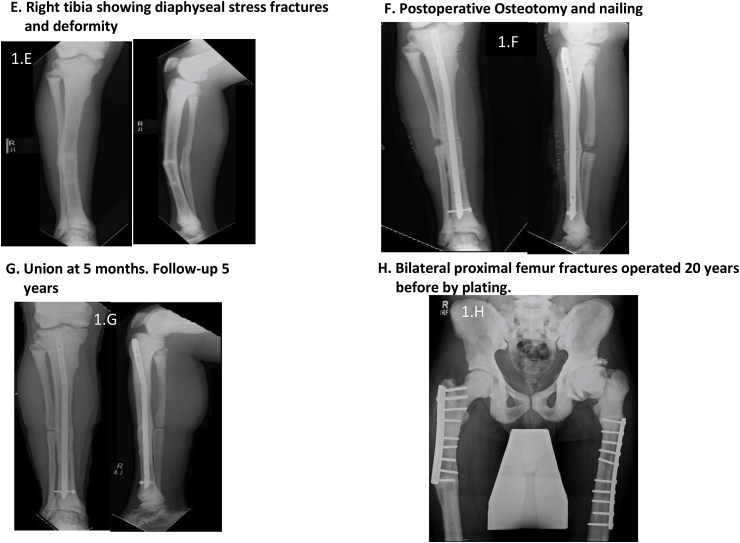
Radiographs of cases 1 and 2 (first three procedures).
